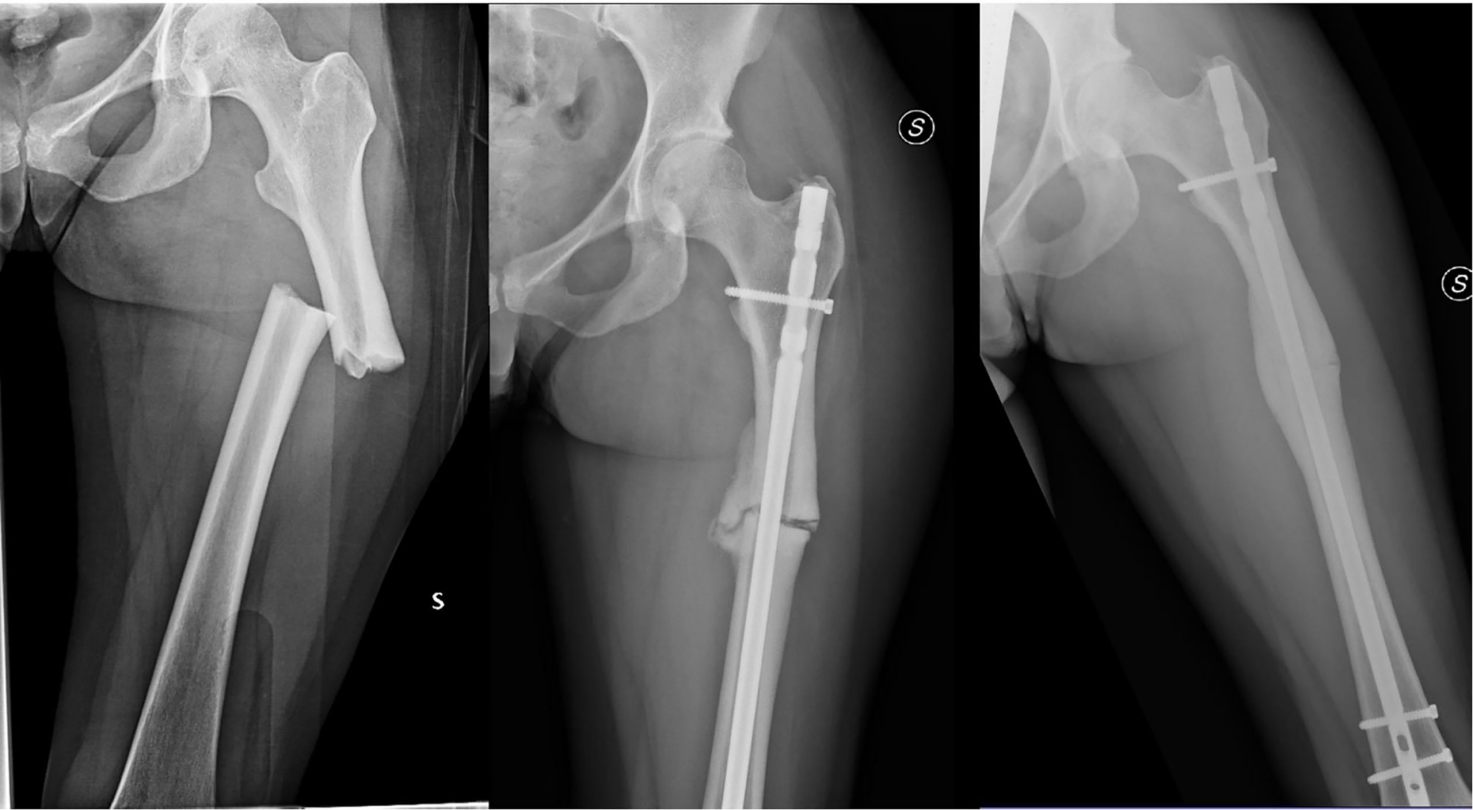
Plain X-ray showing complete, displaced femoral diaphyseal fracture (left panel), reported as an atypical femur fracture (AFF). Patient was treated with open reduction and intramedullary nailing, but bone healing was delayed (plain X-ray after 5 months from surgery, central image). Bony callus eventually fully consolidated after 13 months (right panel).
